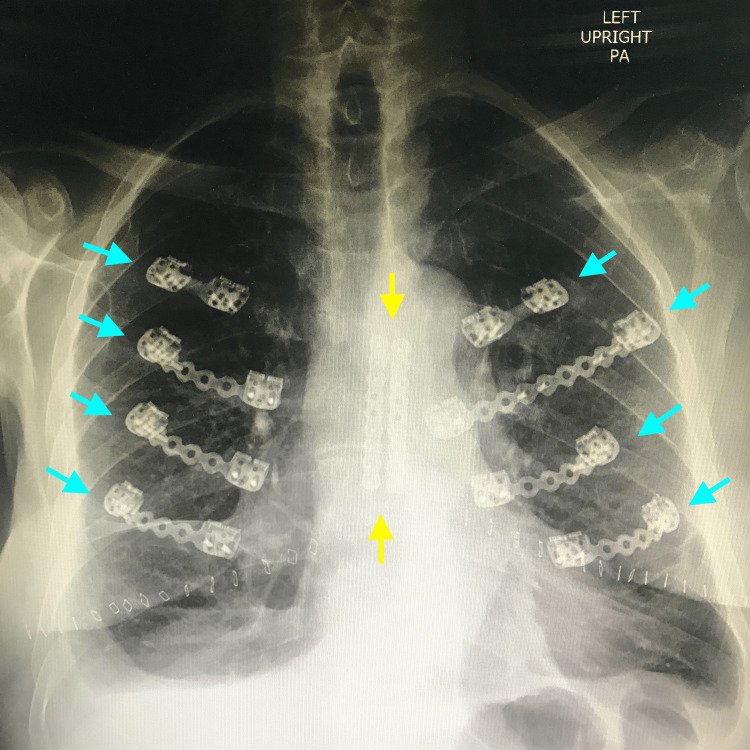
Postoperative radiograph of single stage repair of anterior flail chest wall injury Postoperative radiograph of surgical stabilization of an anterior flail chest wall injury with stabilization of bilateral anterior rib fractures, costal cartilage injuries, and sternal fracture. Blue arrows: stabilization of anterior rib and costal cartilage fractures. Yellow arrows: stabilization of the sternal fracture. The image is from one of the cases treated by the authors.
